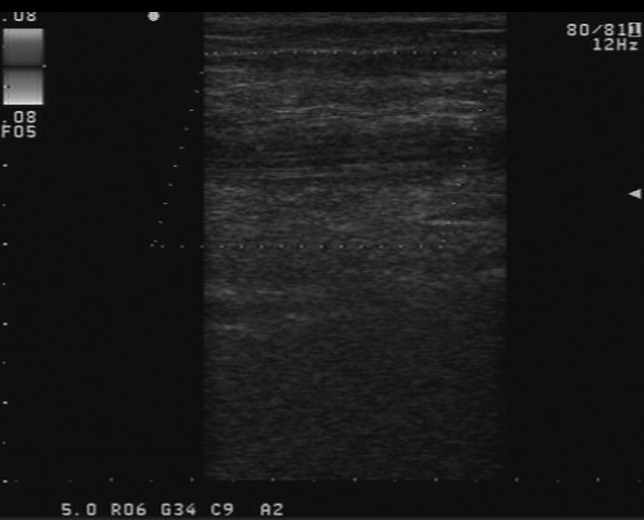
Color doppler ultrasound displays venous thrombosis in the brachial vein.
